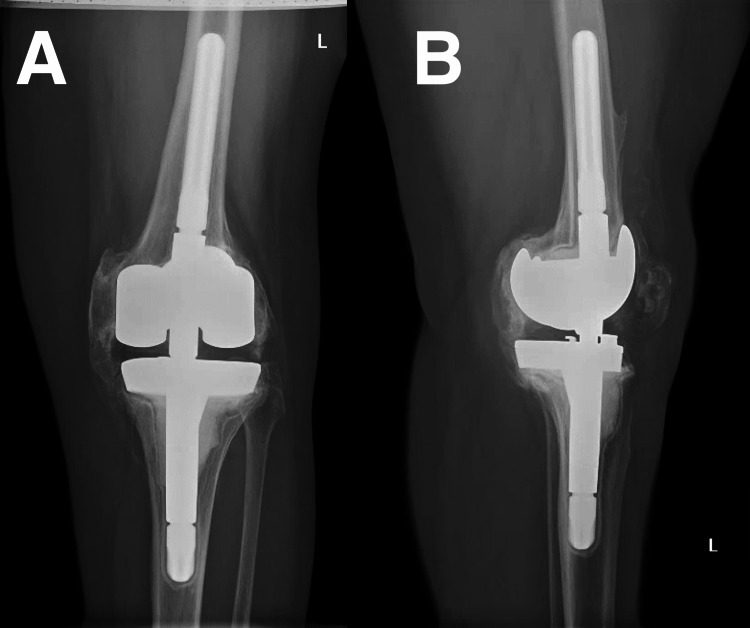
Anteroposterior (A) and lateral (B) radiographs of the left knee demonstrating heterotopic ossification medially, laterally, and posteriorly.
